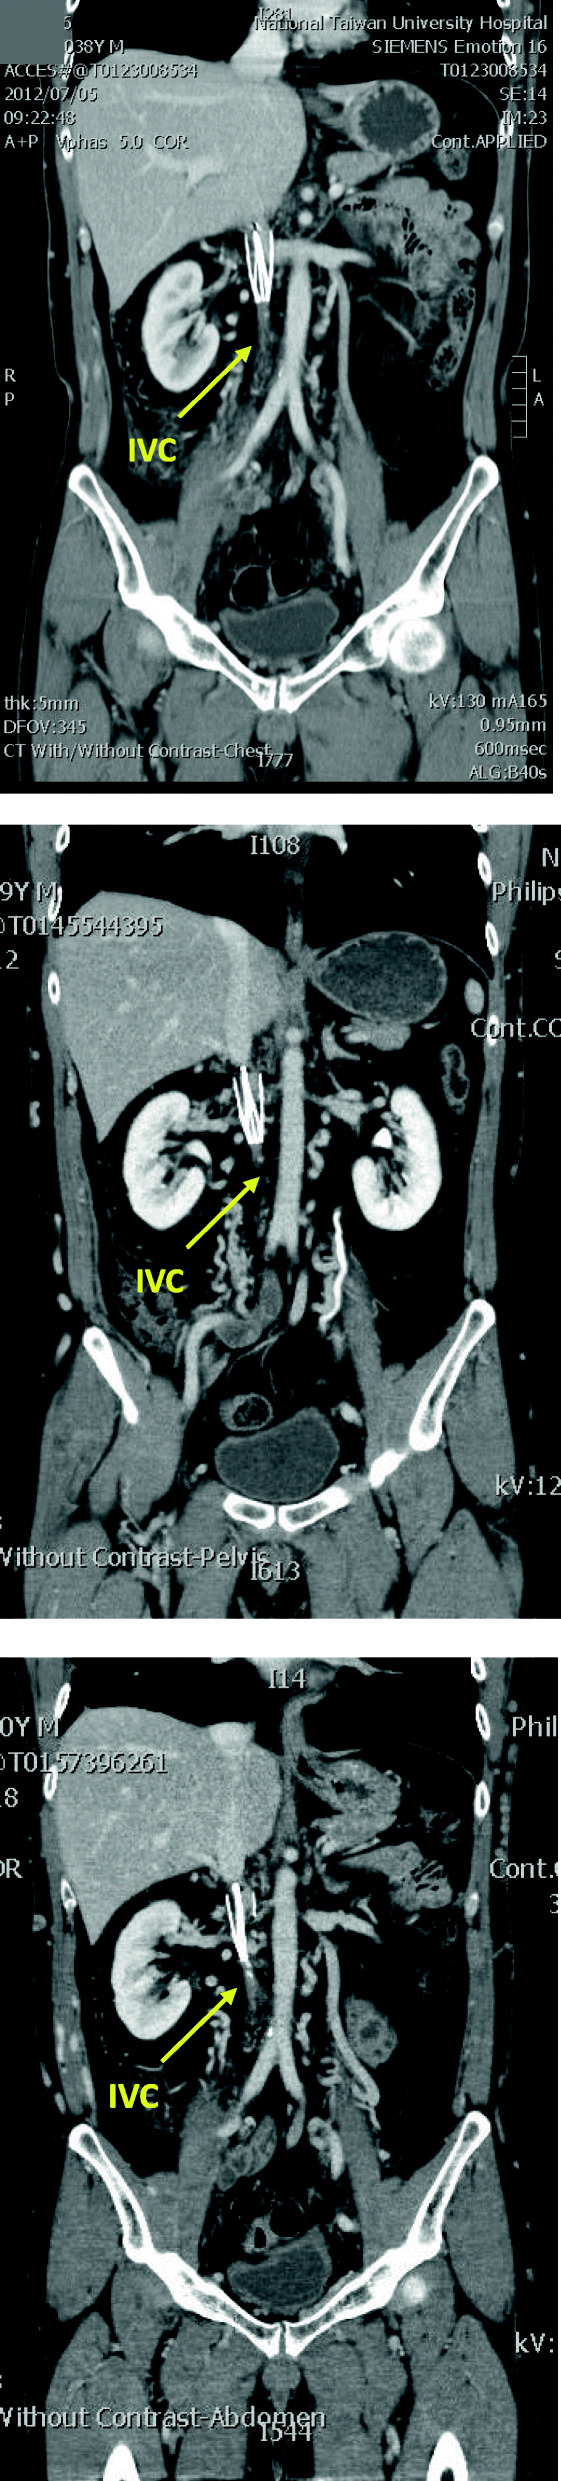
Bilateral lower extremity DVT and IVC thrombosis occurred in a 38-year-old man. (A) Computed tomography demonstrated a filter bearing IVC thrombosis. In the follow-up at 21 months (B) and 33 months (C) later, computed tomography revealed a residual thrombosis. DVT, deep venous thrombosis; IVC, inferior vena cava
